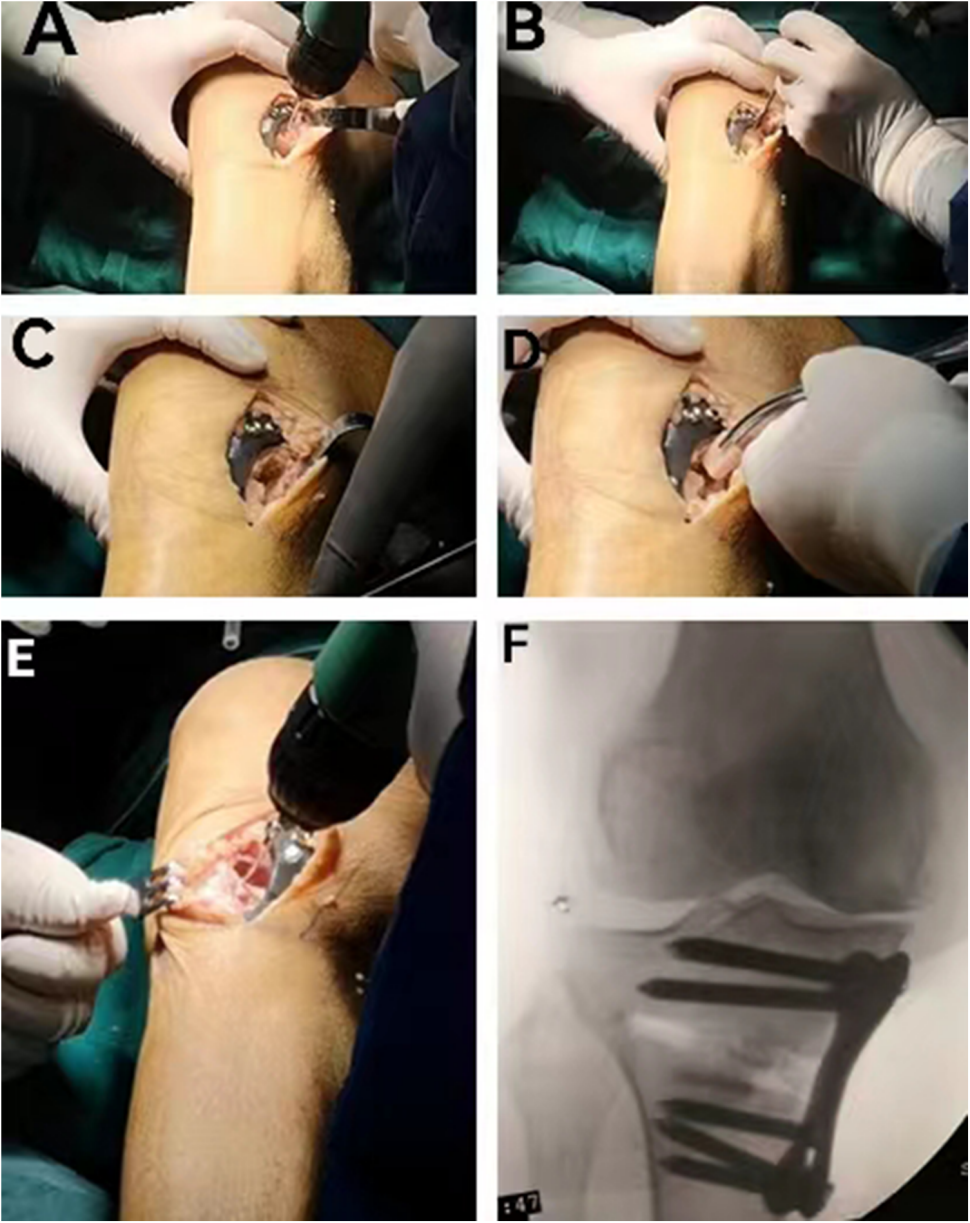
The procedure of the OBFT-FT. (A) A Kirschner-wire 1.0 mm in diameter was used to conduct the U-shaped osteotomy, (B) the remaining bone tissue was chiseled out with an osteotome for about 6 mm, (C) the orthotopic bone flap was performed, (D) half of the fibula was implanted into the gap, (E) contralateral U-shaped osteotomy, and (F) the intraoperative radiograph after OBFT-FT.
